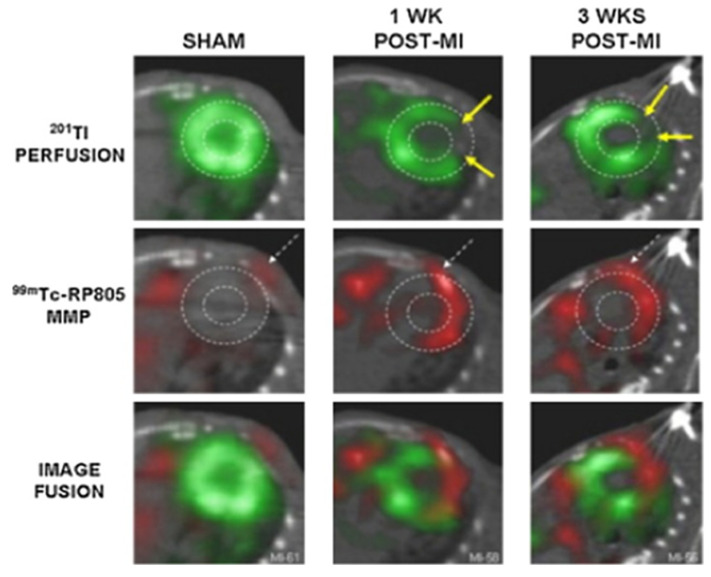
^201^Tl perfusion imaging, ^99m^Tc labelled MMP imaging, and fused images, 1 and 3 weeks after myocardial infarction using SPECT/CT [86].
